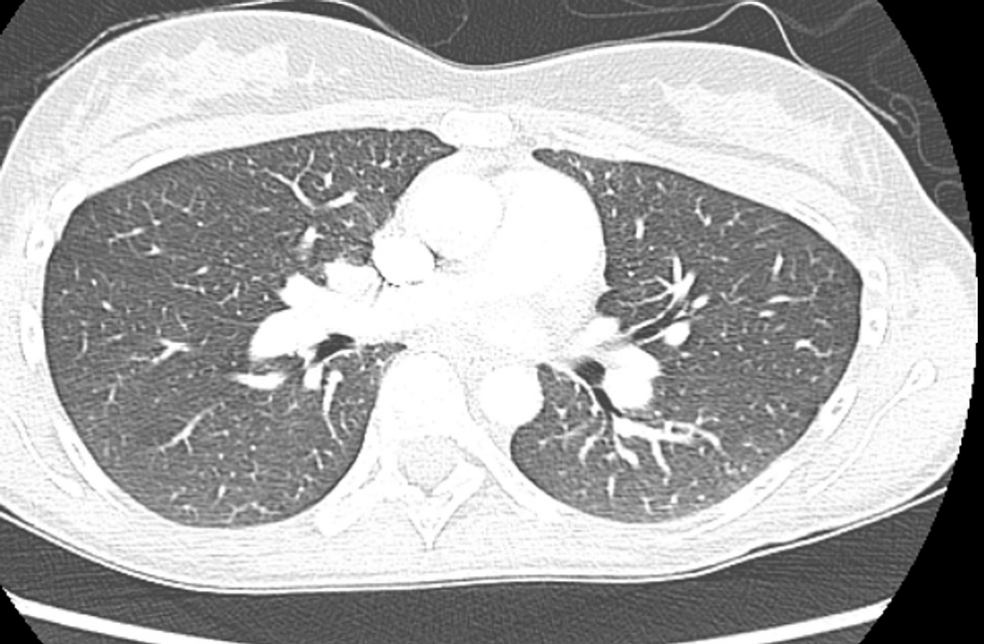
CT Angiography of the chest did not show any central, lobar, segmental, or proximal sub-segmental pulmonary embolism
